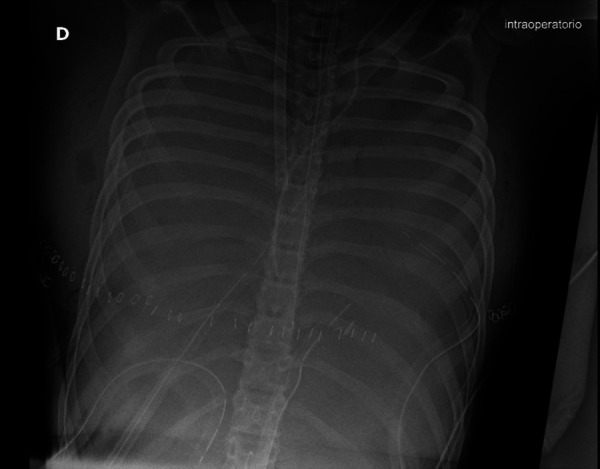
Intraoperative x-ray chest showed an ARDS syndrome.
